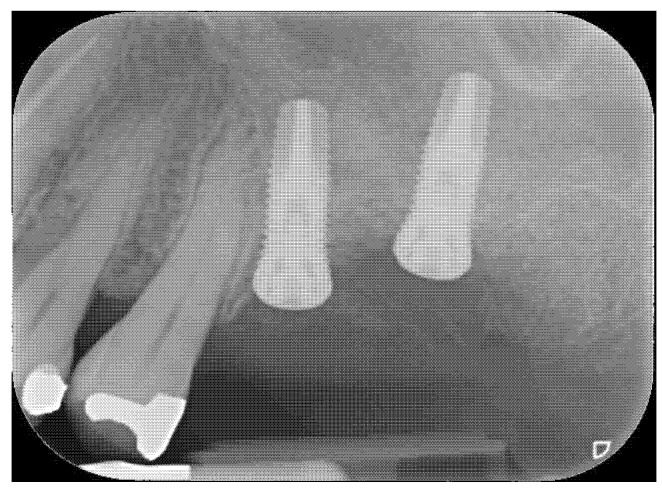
Radiographic control at 5 years.
